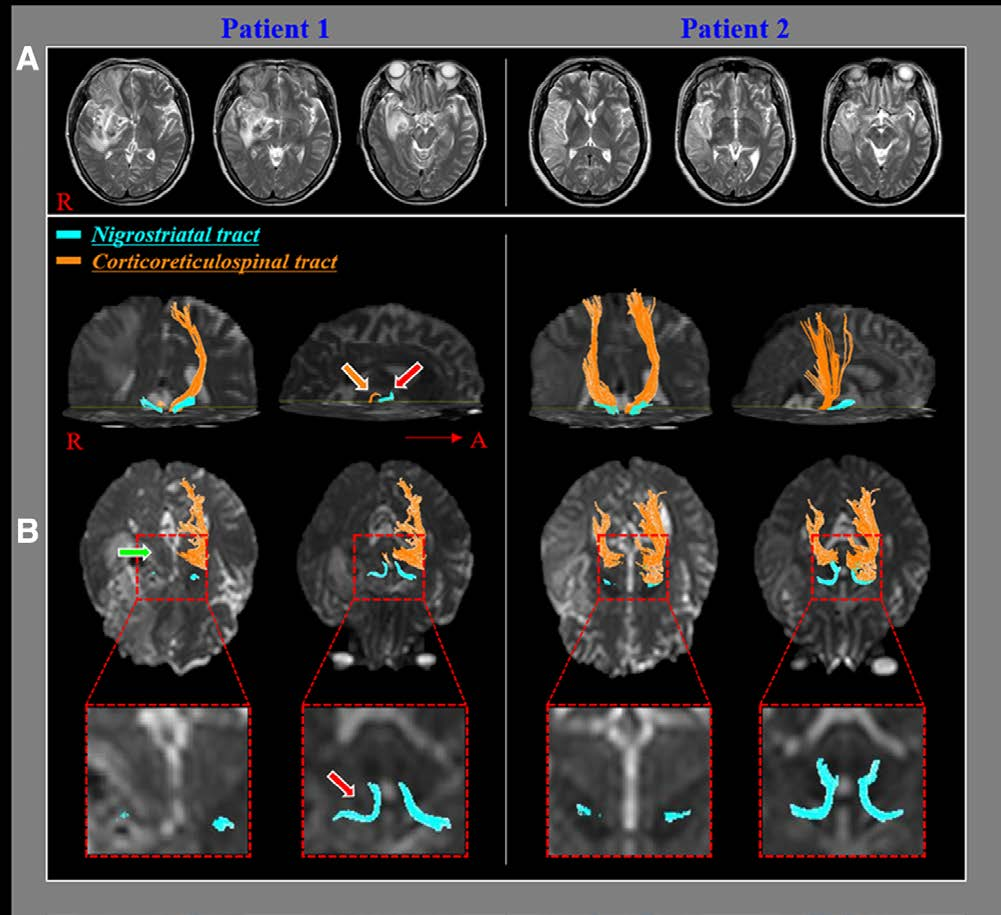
Results of diffusion tensor tractography for the nigrostriatal tract (NST) and the corticoreticulospinal tract (CRT). (A) T2-weighted brain magnetic resonance images at the time of diffusion tensor imaging scanning in representative patients with a middle cerebral artery territory infarction (patient 1:51-year-old female with functional ambulation category [FAC] score 2 at the chronic stage, patient 2: 67-year-old female with FAC score 5 at the chronic stage). (B) The ipsilesional NST (red arrow) and the ipsilesional CRT (orange arrow) at the early stage in patient 1 have a lower tract volume than those in patient 2. The ipsilesional CRT (green arrow) at the early stage in patient 1 shows discontinuation at the lesion. By contrast, preservation of the integrity of the ipsilesional CRT at the early stage is observed in patient 2.
